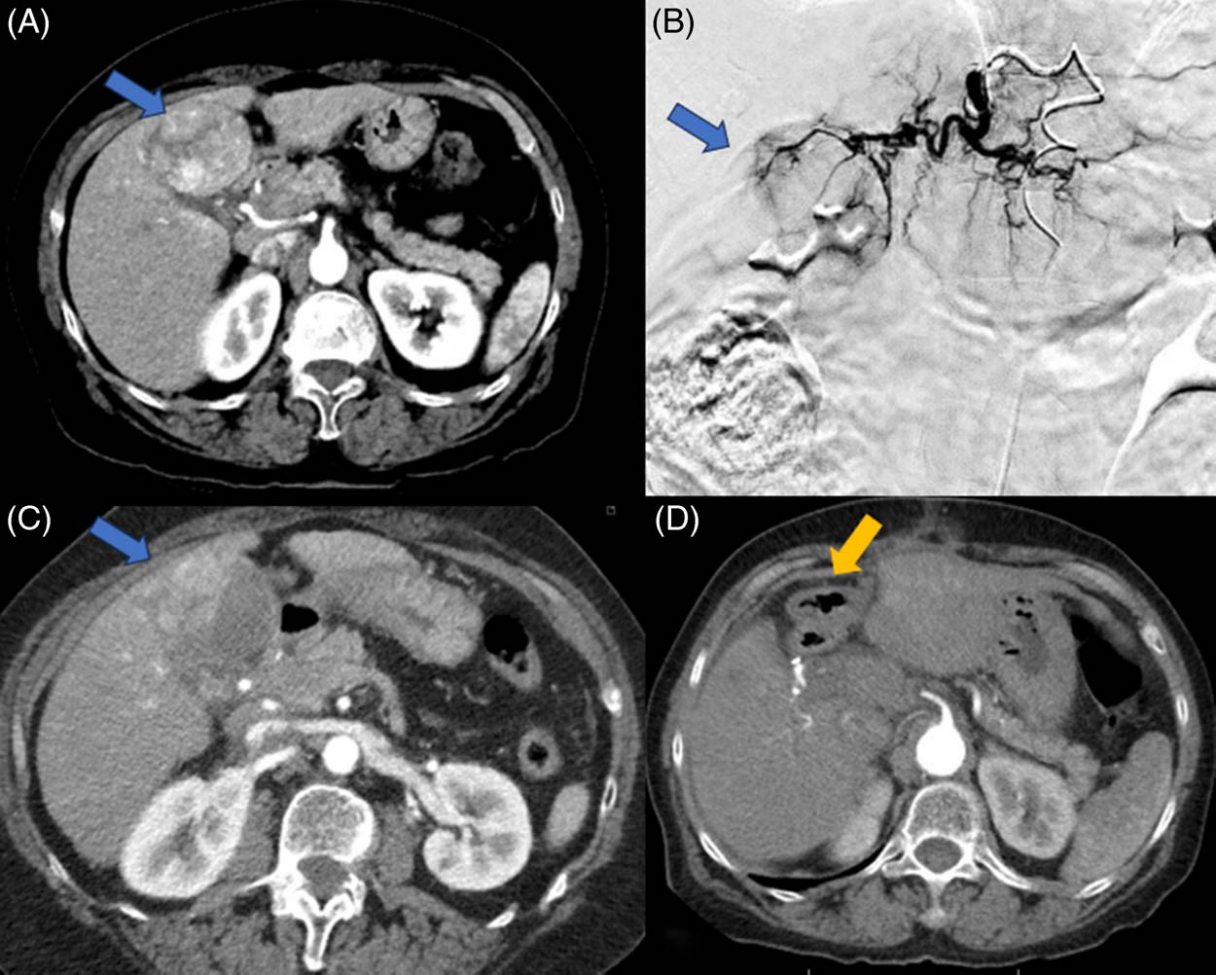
Patient with well-compensated hepatitis C cirrhosis and HCC situated in the central liver (blue arrow). (A) CT demonstrated arterial phase enhancing segment IVB tumor measuring 5.1 × 4.7 cm. (B) Catheter angiogram during selective radioembolization via segment IVB branch. (C) Postembolization CT shows partial response with the enhancing portion measuring 4.6 × 2.6 cm. (D) Patient underwent partial hepatectomy of segment IVB/V with a negative margin (yellow arrow: surgical bed).
